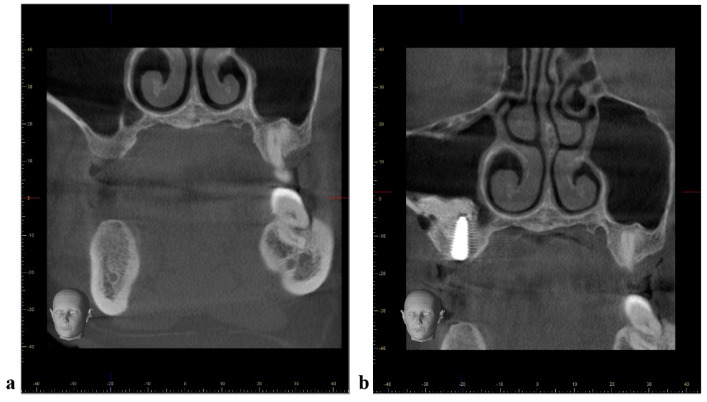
Management of antero-posterior septa. (a) CBCT coronal view of the left maxilla with a antero-posterior oriented septa. (b) CBCT coronal view after maxillary sinus floor augmentation with simultaneous dental implant placement (one window from lateral approach)—the implant prosthetically driven position did not require augmentation of the medial compartment of the sinus.
